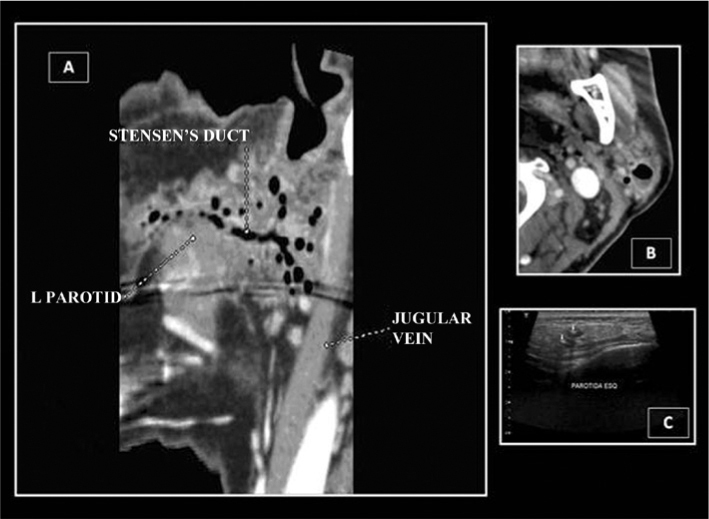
Radiological aspect of the pneumoparotitis seen through CT scan (A and B) and ultrasound (C).
